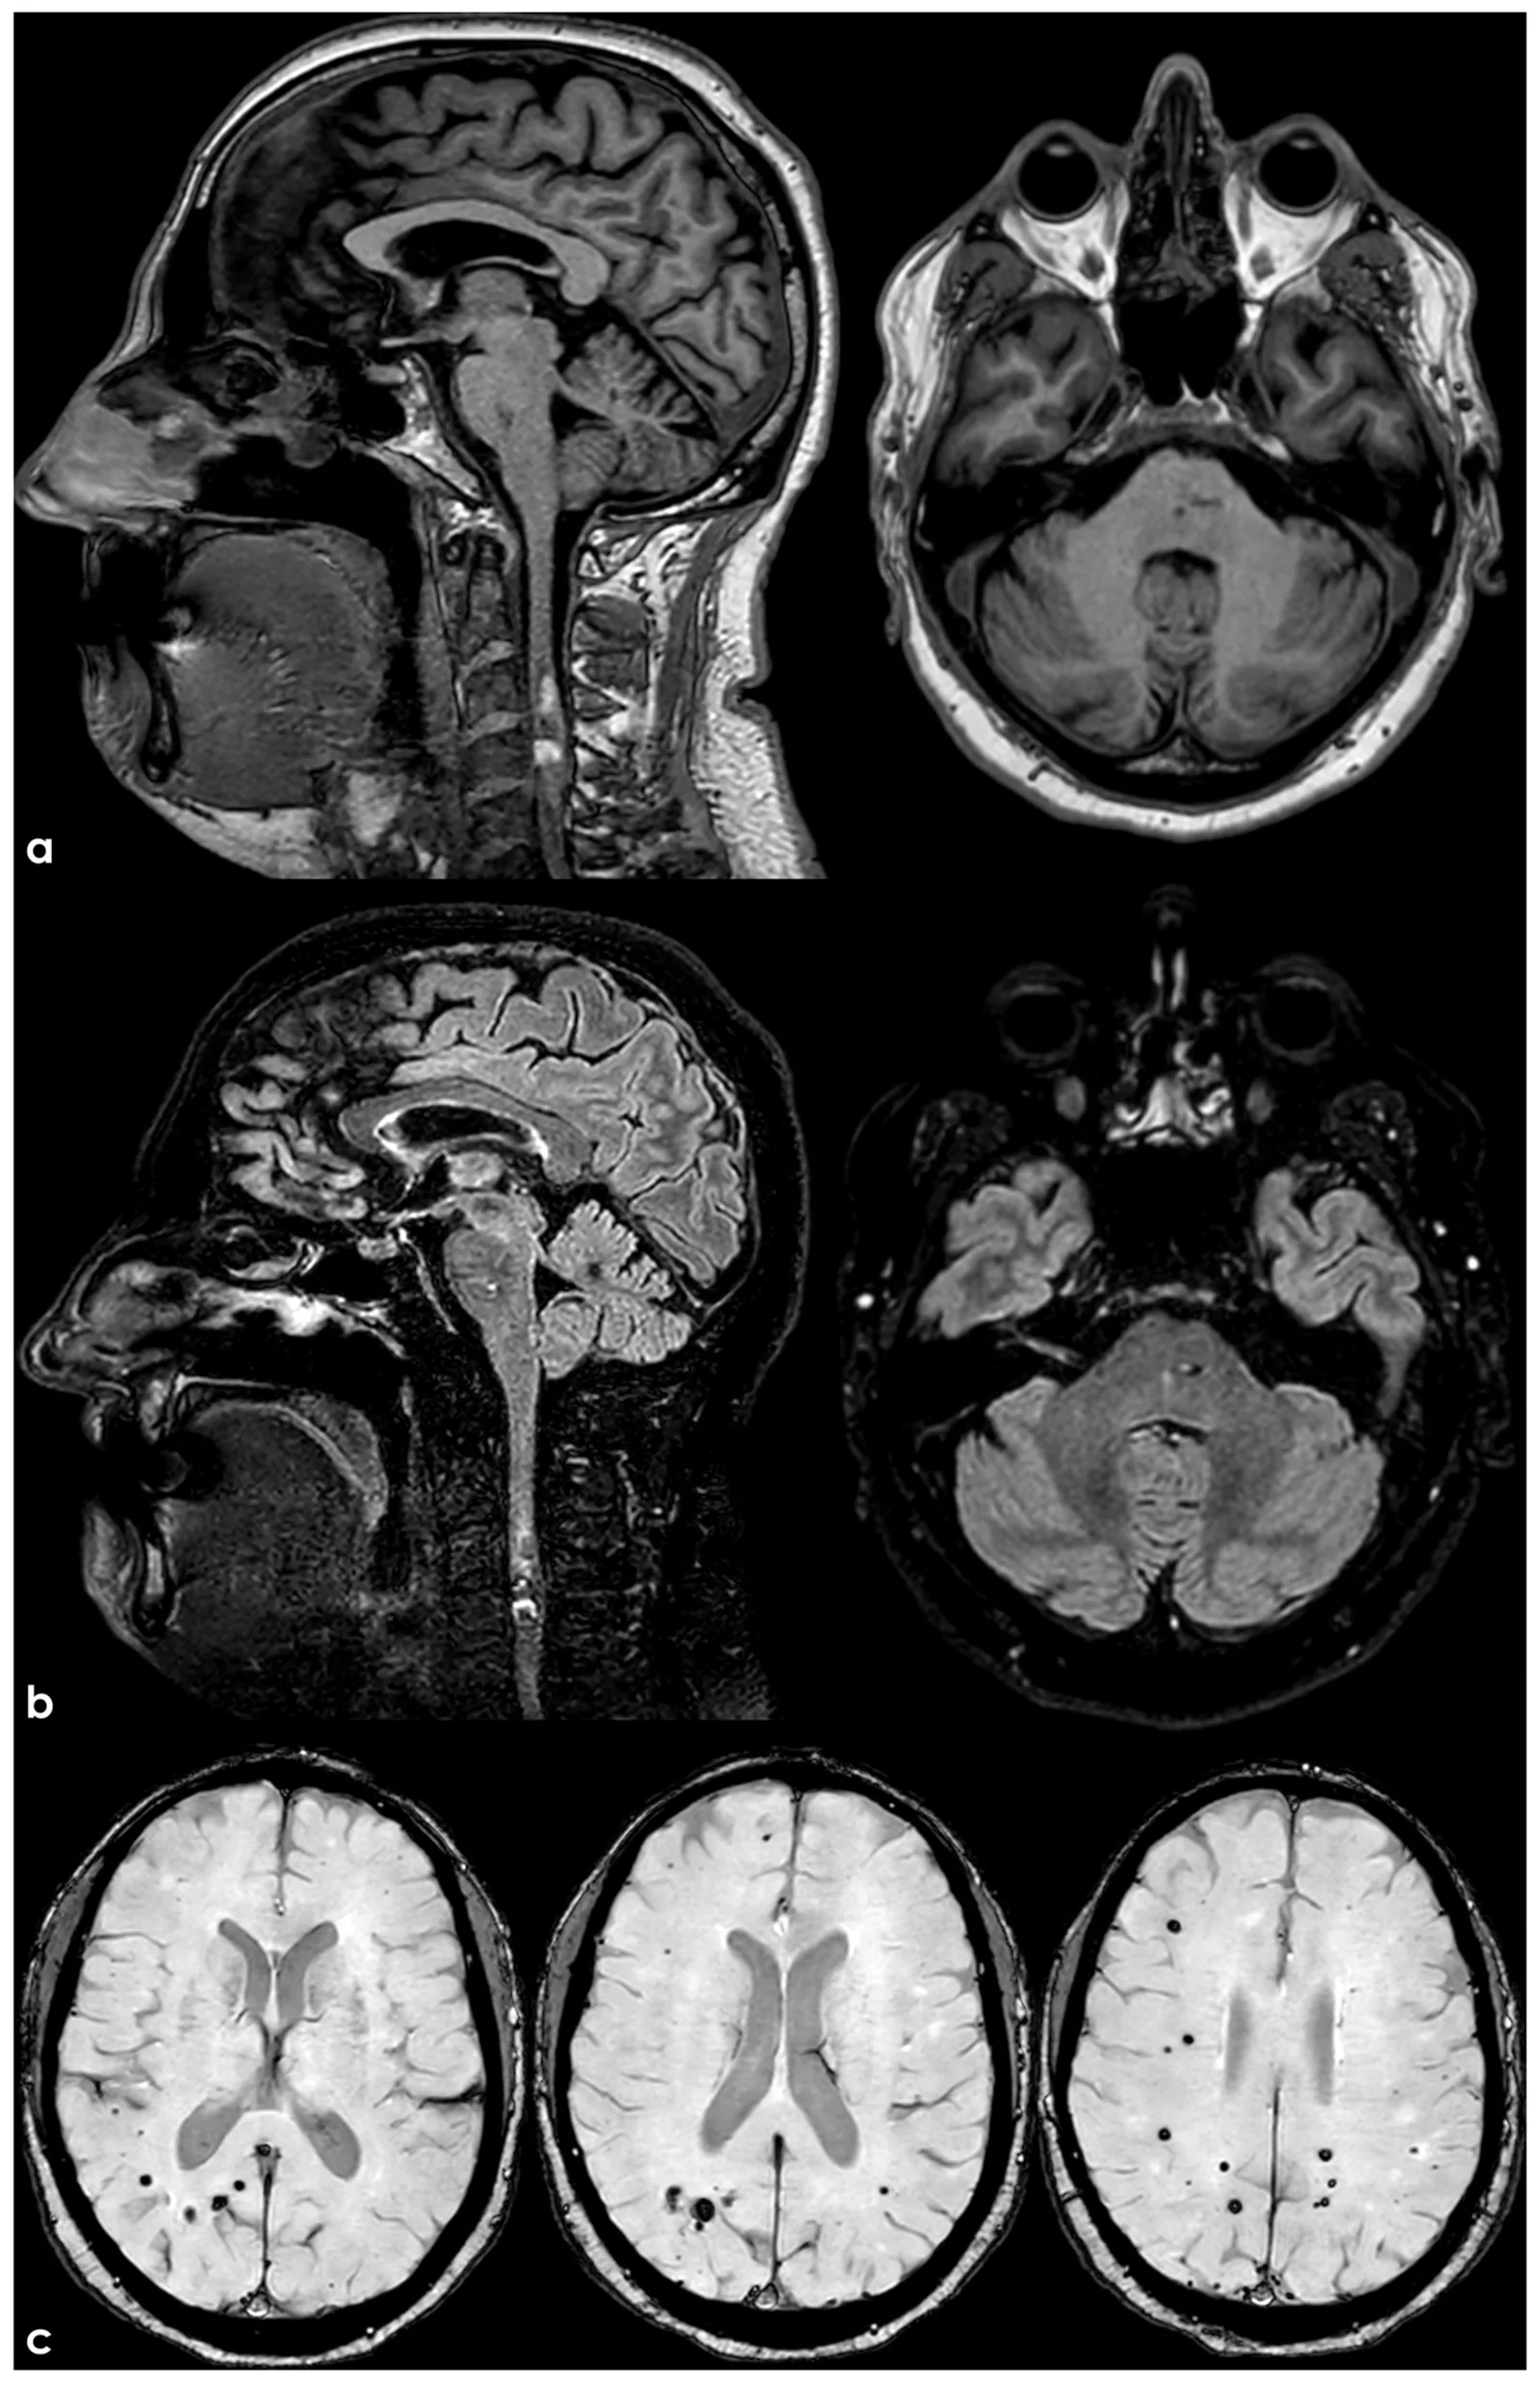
Brain MRI of a young patient complaining the subacute occurrence of paresthesias on the left side with head sparing. The MRI was performed at 3 weeks from symptoms onset. (Panel a): 3D T1W sequence reconstructed in sagittal (left) and axial plane (right), showing a left hemipontine hypodense lesion and two cervical tumefactive hyperintense intra-axial lesions. (Panel b): Same findings on sagittal (left) and axial 3D T2-Fluid Attenuated Inversion Recovery (FLAIR) sequence, showing an hyperintense signal in the cervical spinal lesions. (Panel c): SWI in axial view showing multiple rounded hypointensities suggesting CCMs (Zabramski 1 and 4).
